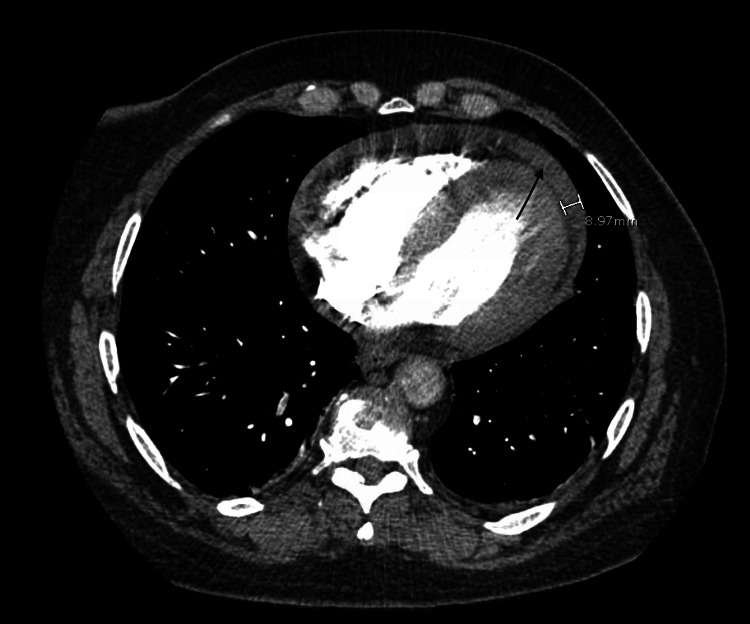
CT of the chest with IV contrast. The axial section shows a pericardial effusion marked by an arrow. CT: computed tomography; IV: intravenous
